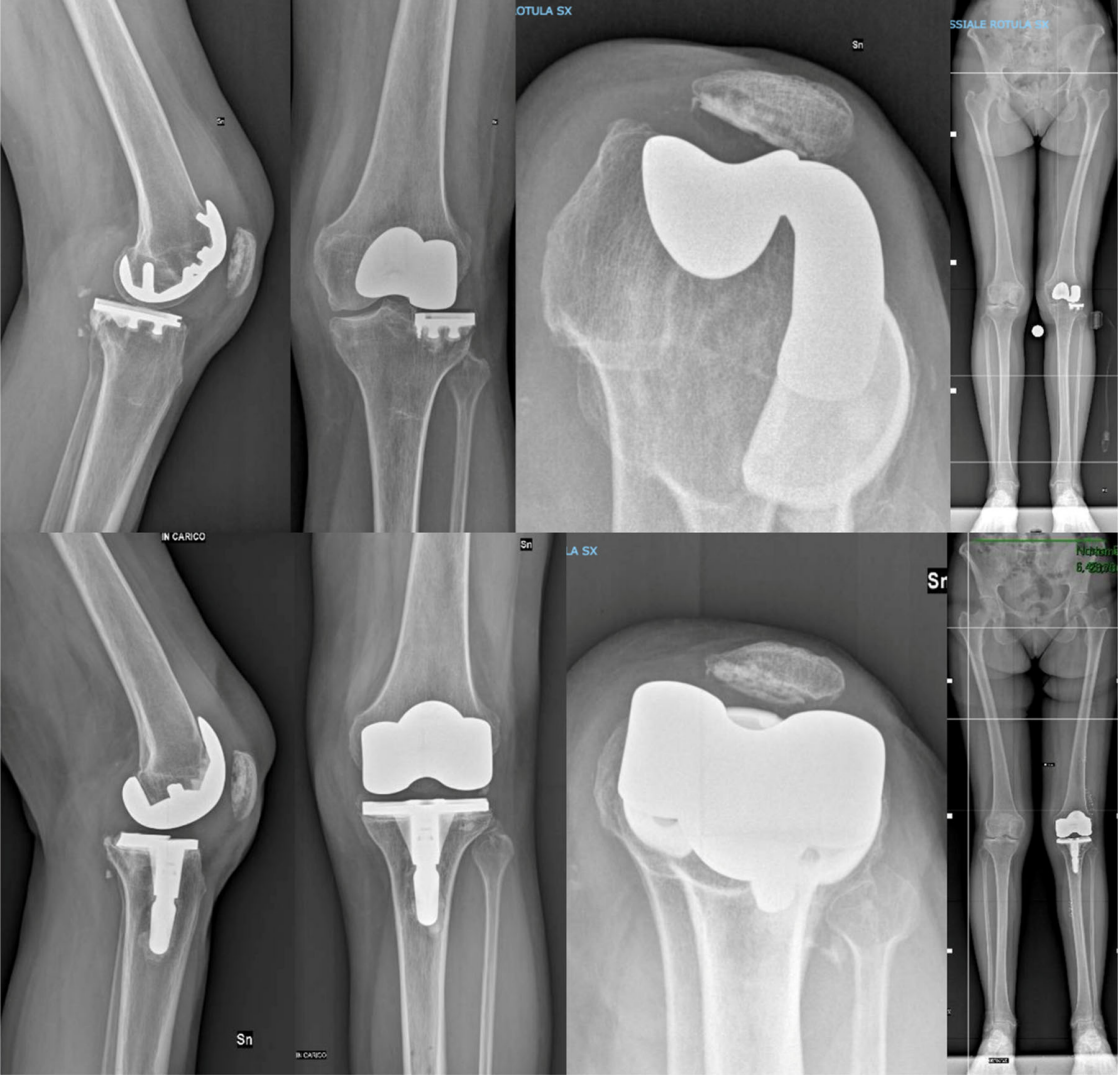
Preoperative and final follow‐up radiographic evaluation of a patient with a lateral unicompartmental and patellofemoral implants, revised using the Rosa Knee System (Zimmer Biomet).
